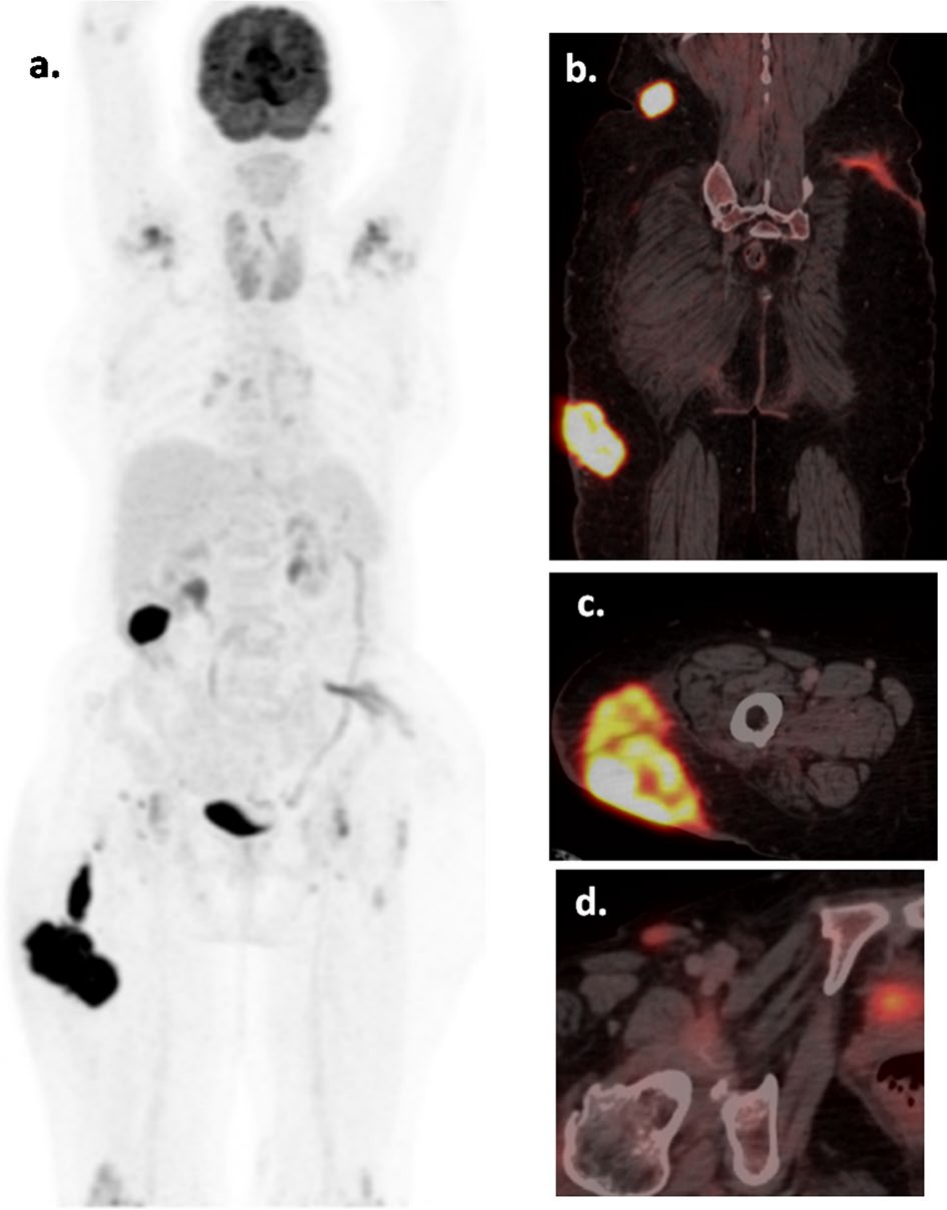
Baseline (staging) F-18 FDG PET/CT images, maximum intensity projection (MIP) image a showing multiple cutaneous and subcutaneous lesions in right lumbar–gluteal (b) and upper thigh (c) regions, along with prominent right inguinal lymph node (d)
